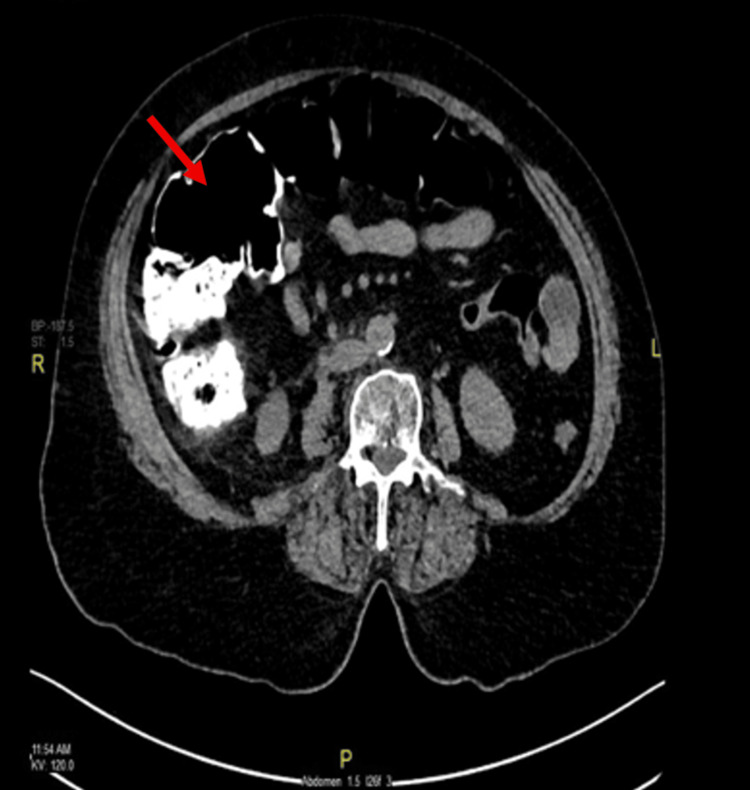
Contrast-enhanced CT abdomen demonstrating features consistent with cecal bascule. Axial CT image of the abdomen revealing a massively distended cecum (indicated by the red arrow) displaced from its normal anatomical position in the right lower quadrant and folding upward, highly indicative of a cecal bascule.
